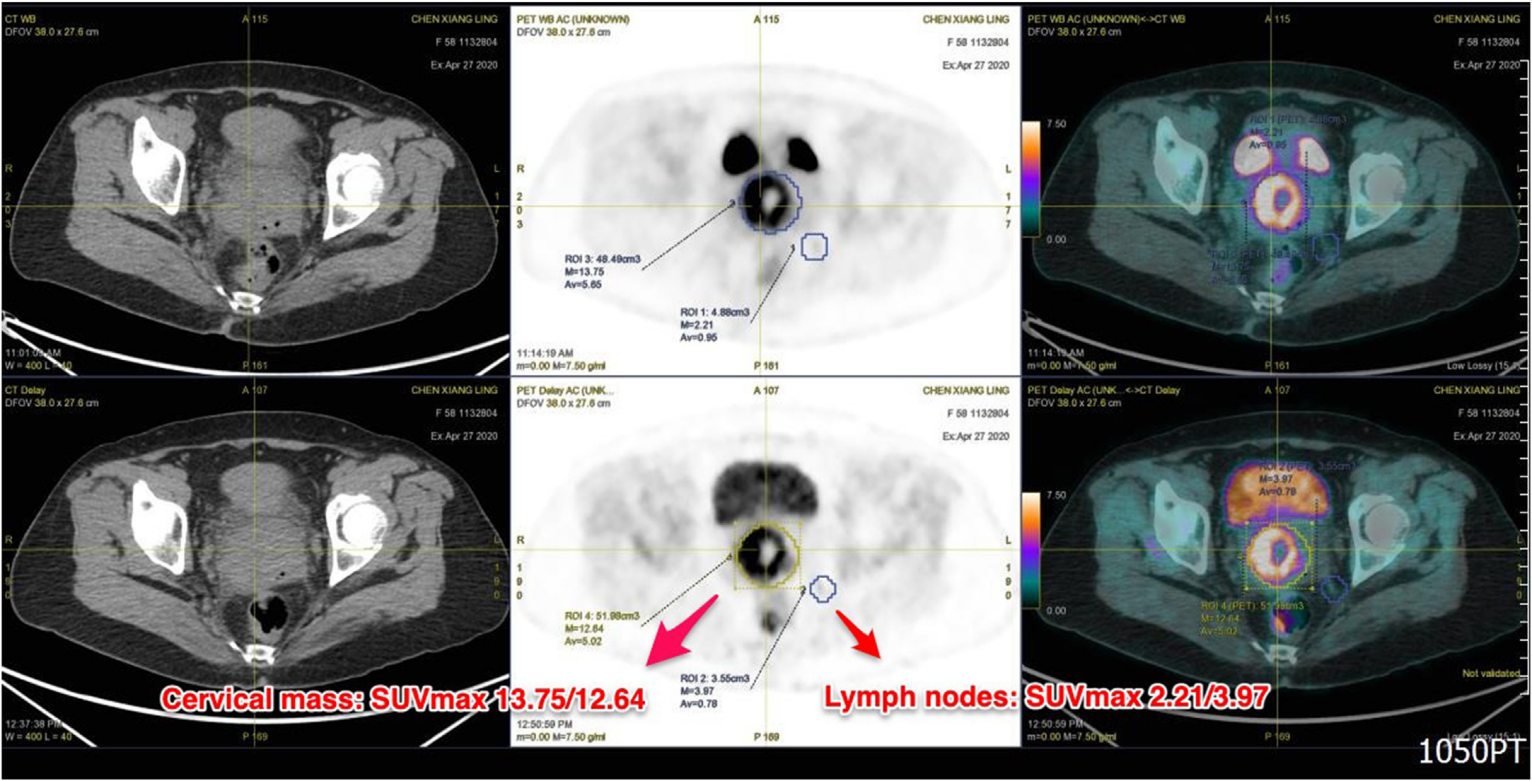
PET/CT with FDG revealed an FDG-avid cervical mass, with invasion of two lymph nodes at the left pelvic cavity.
